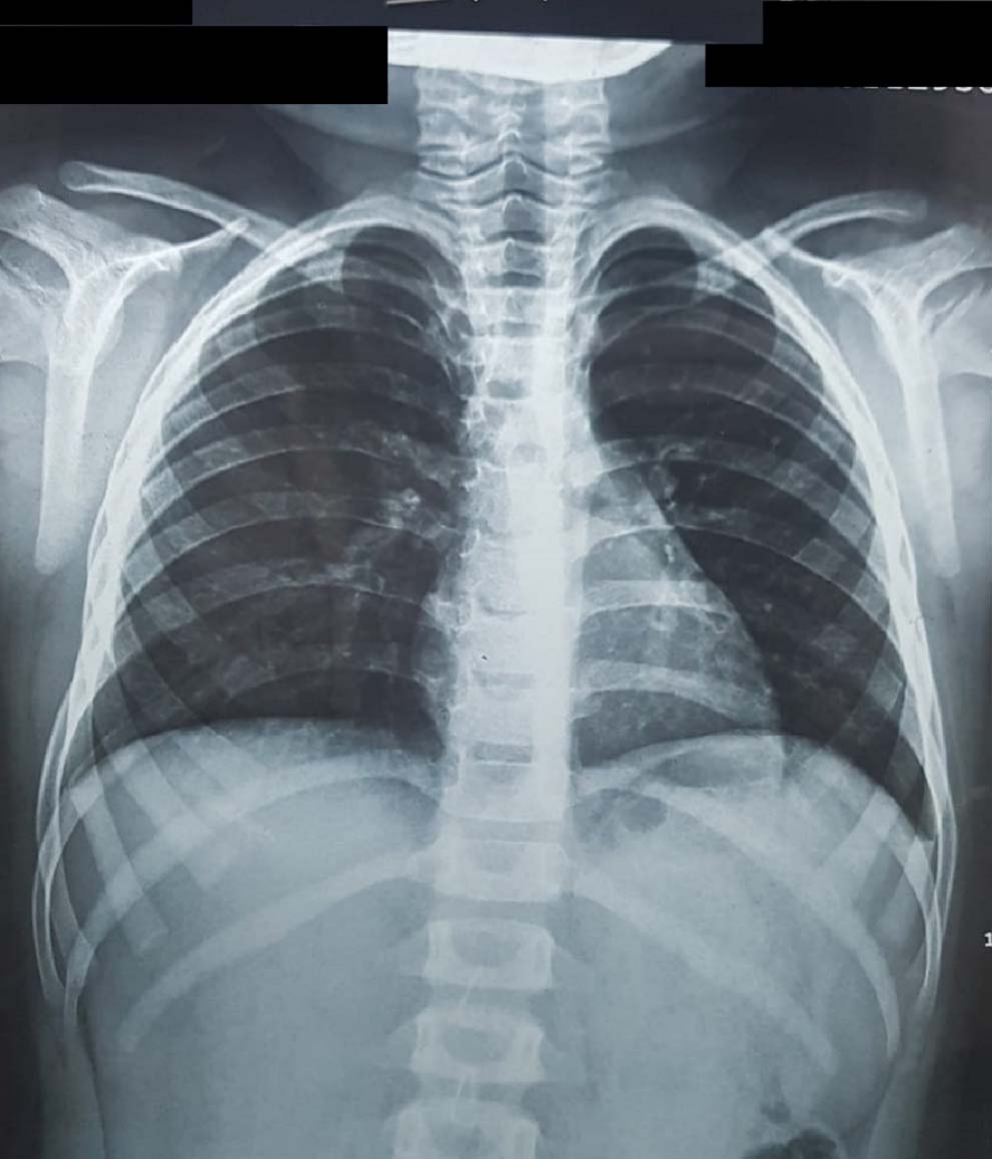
Chest x-ray was unremarkable, showing no signs of tuberculosis.
